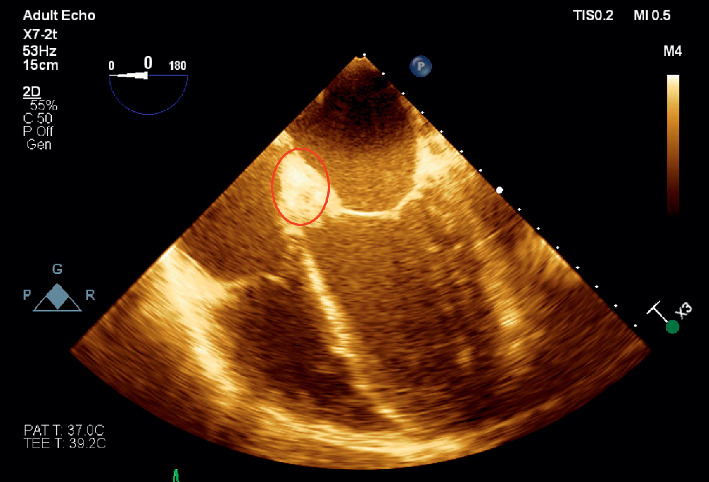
Transesophageal echocardiogram showing a 4-chamber view. Paravalvular abscess is circled.
